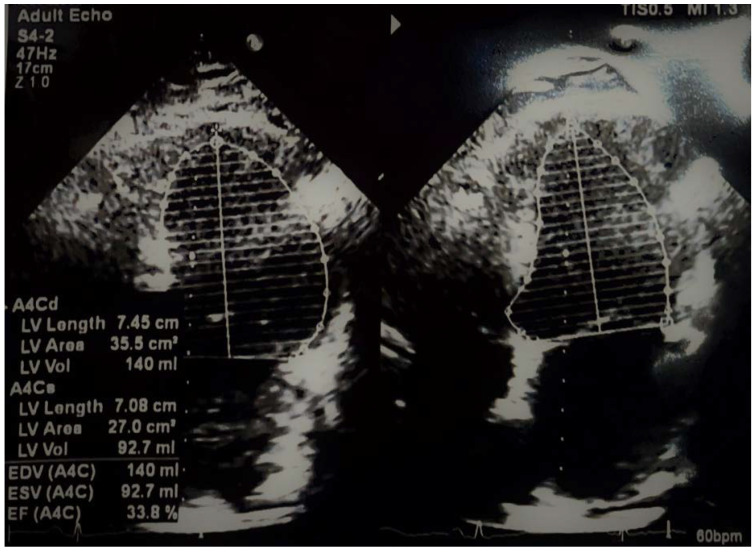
Apical 4-chambers (A4C) echocardiography: moderately decreased left ventricular ejection fraction (LV, left ventricle; EDV, end-diastolic volume; ESV, end-systolic volume; EF, ejection fraction).
